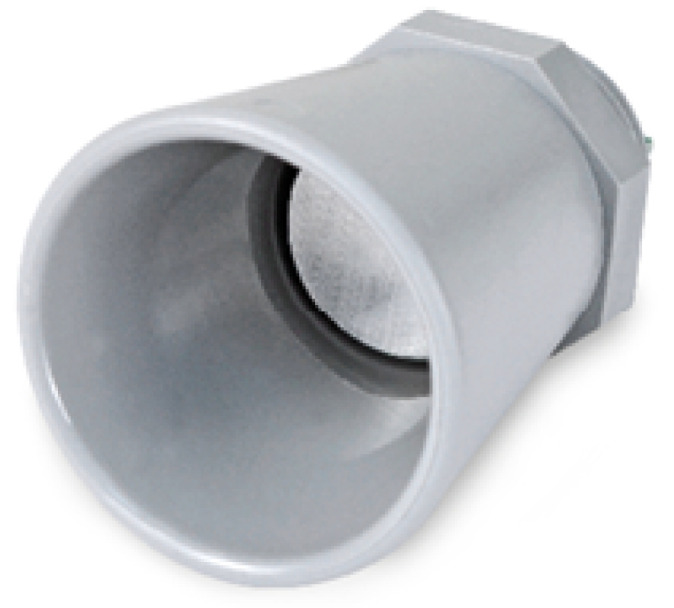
Ultrasonic I2CXL- MaxSonar^®^-MB7040 from Max- Botix^TM^ sensor.
